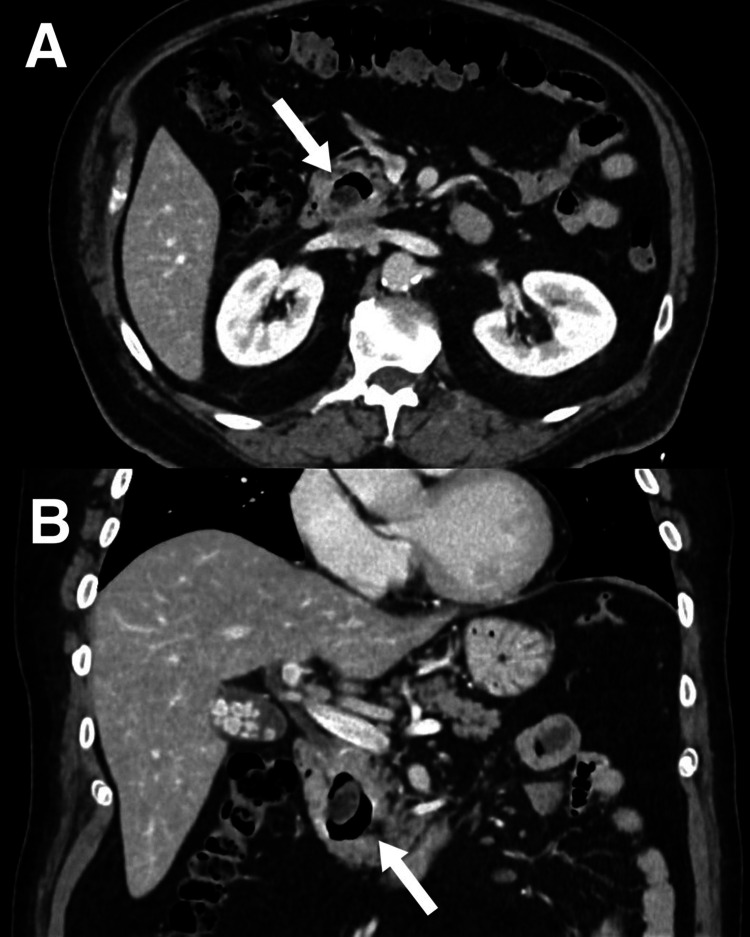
Axial (A) and coronal (B) IV contrast-enhanced CT of the abdomen showing a duodenal diverticulum, noted adjacent to the distal end of the common bile duct. The diverticulum measures 2.2 x 1.5 cm.
